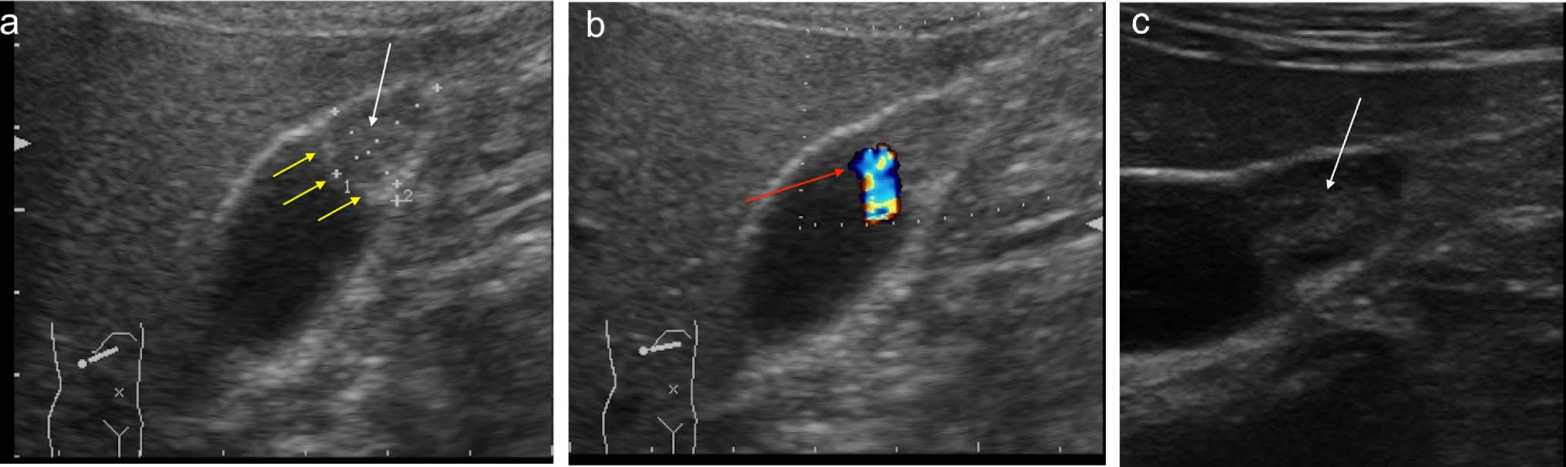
a Ultrasound image shows a segmental type of GA that involves a larger portion of the gallbladder wall, the bottom, and the distal third of the body. The affected part appears hypoechoic (white arrows), with hyperechoic cholesterol crystals (yellow arrows). b The hyperechoic cholesterol crystal of the segmental type of GA generating twinkling artifacts on the colour Doppler exam (red arrow). c The segmental type of GA visualised with a multifrequency linear probe (white arrow)
